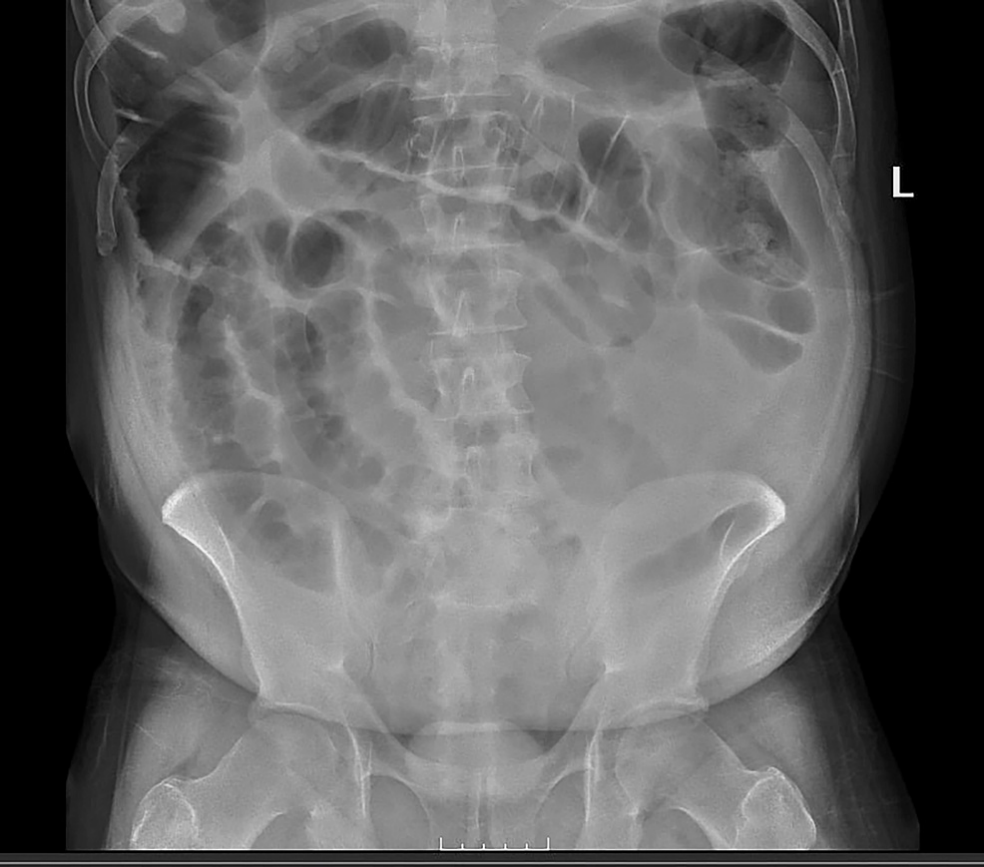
Abdominal X-ray showed diffuse ileus.
